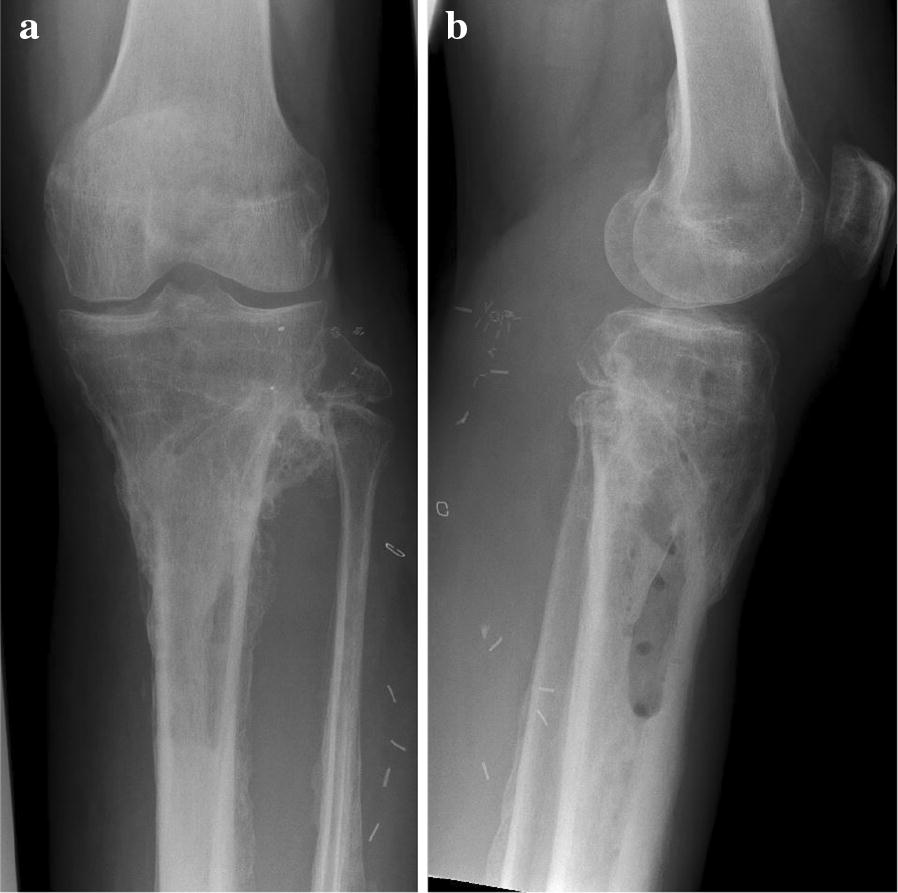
Osseous consolidation 12 months after initial trauma. a X-ray a.p. view and b X-ray lateral view
